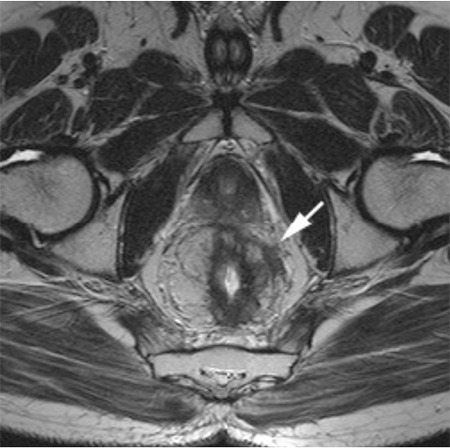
29-year-old man with pT4N1 rectal cancer. First observerstaged the tumor as T3N2, second observer staged as T4N2 in thepreoperative MR imaging. Two observers accurately predicted CRMinvolvement. T2-weighted para-axial MR image shows the tumor withmesorectal fascia invasion at the left side
